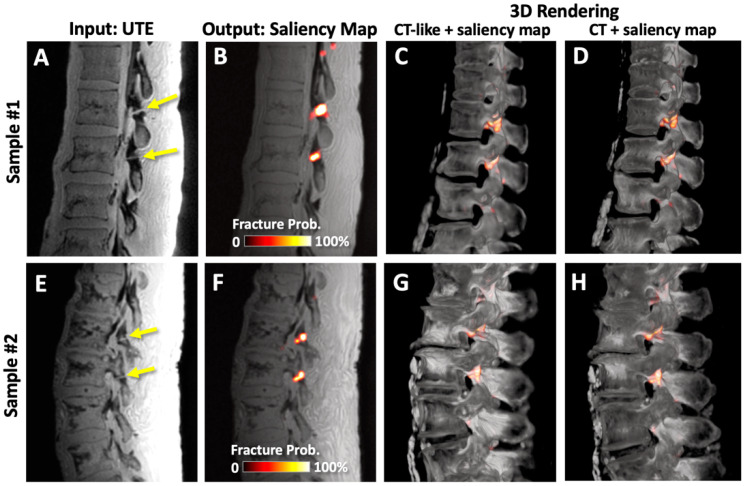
Saliency mapping model takes in (A,E) UTE images and outputs saliency colormaps. (B,F) Saliency maps overlaid onto UTE images show colored areas covering the pars defects. Saliency maps can also be fused with (C,G) CT-like images to create 3D fused rendering that highlights pars defects. Note the high similarity between the fused rending using (C,G) CT-like vs. (D,H) CT images.
